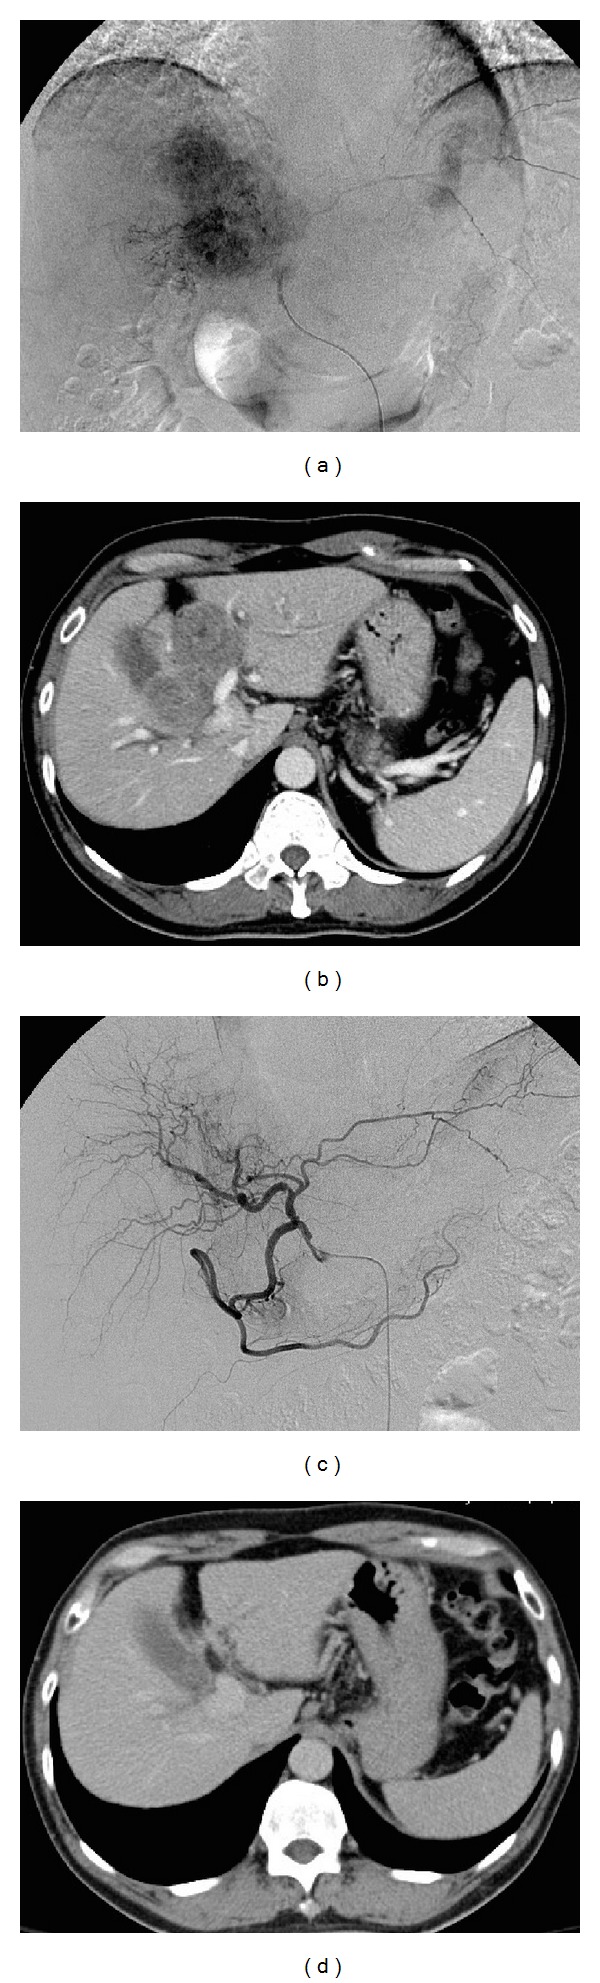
(a) Angiogram of the hepatic artery obtained before intra-arterial chemotherapy. (b) Computed tomography image of the liver obtained before intra-arterial chemotherapy. (c) Angiogram of the hepatic artery obtained after two cycles of intra-arterial chemotherapy. (d) Computed tomography image of the liver obtained after two cycles of intra-arterial chemotherapy.
